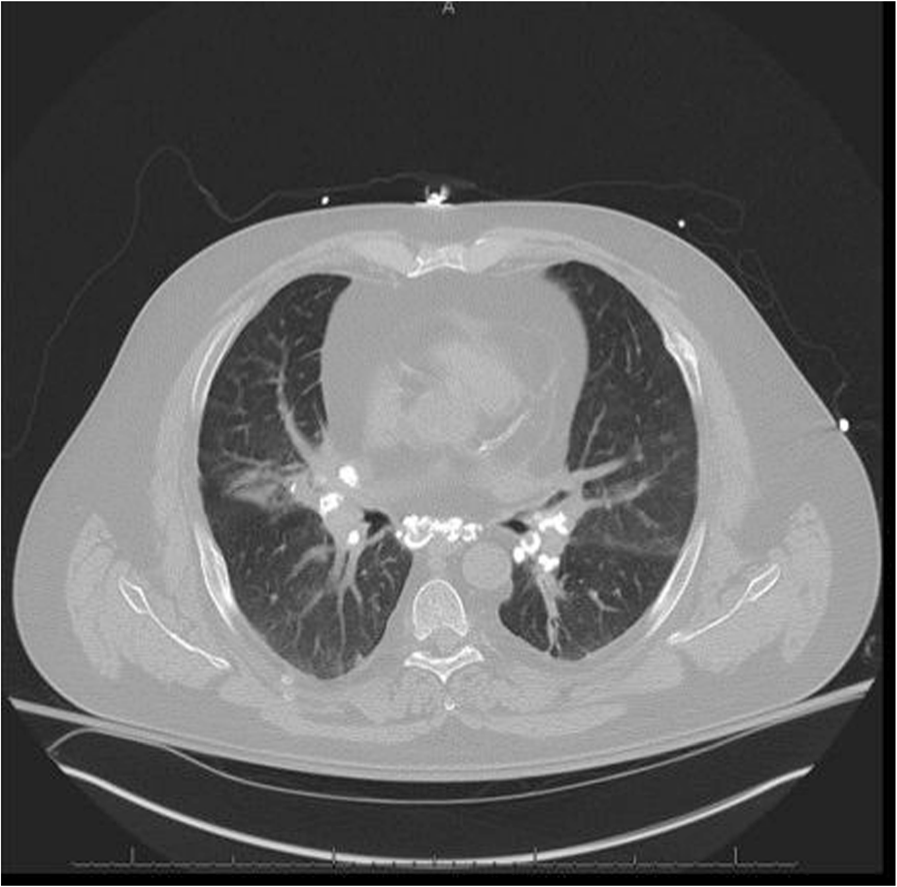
Noncontrast-computed tomography scan. This screenshot of the chest computed tomography scan obtained at admission shows pulmonary nodules and calcified mediastinal and hilar lymphadenopathy, which can be found both in sarcoidosis and *Histoplasma* infections.
